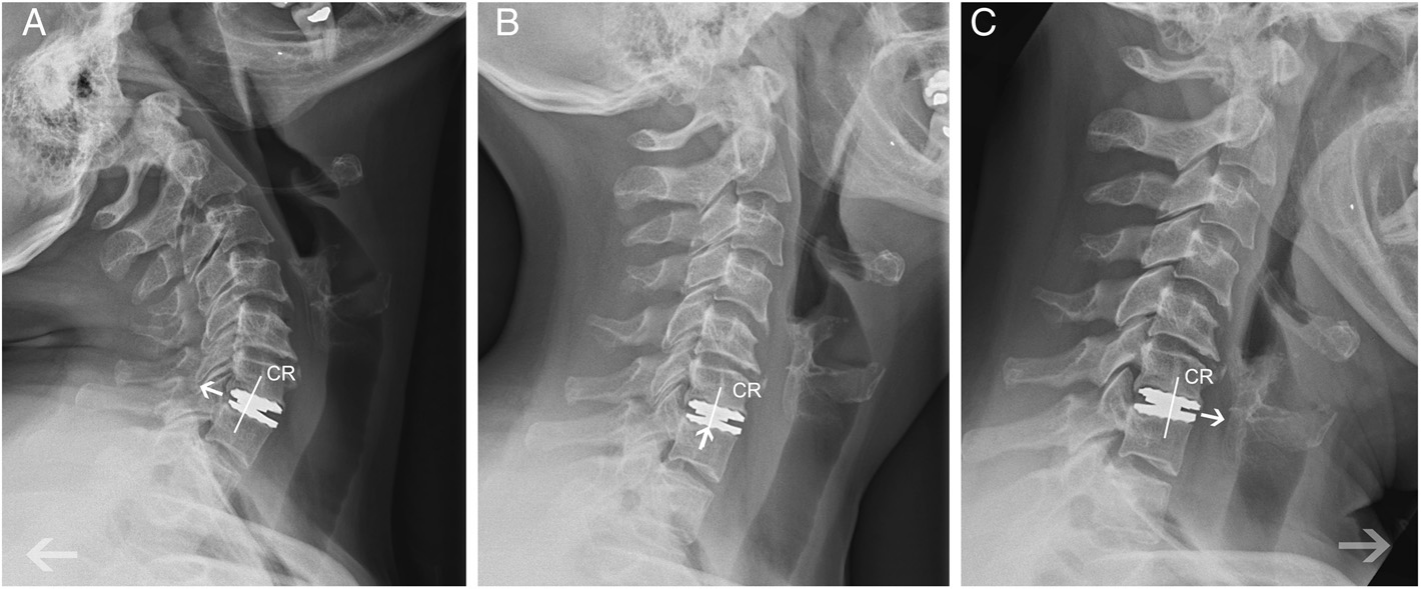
Flexion (a), neutral (b) and extension (c) x-ray showing the successful operation – especially the variable mechanical centre of rotation (CR) of the device moving forward and backward (white arrows) - of a ROTAIO cervical disc prosthesis at the level C6-C7 in a 52 years old female patient, 6 month after implantation
